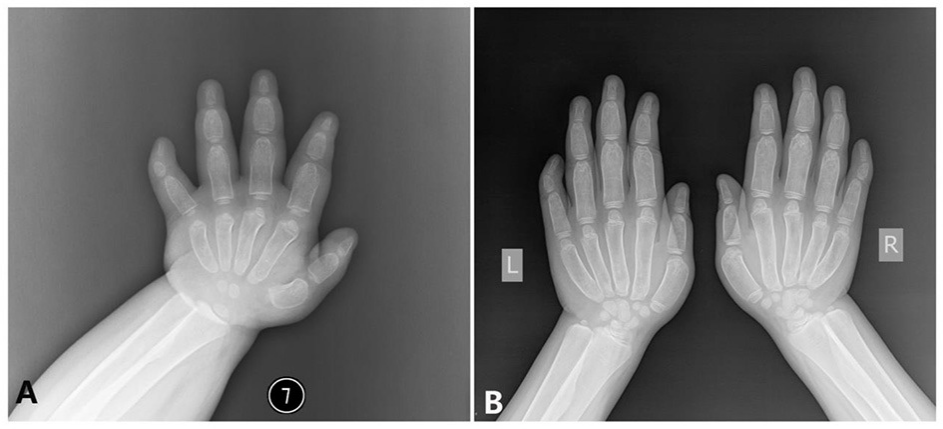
The x-ray radiography of the hands [shows short and wide phalanges and deformable bones; (A) age 1 year and 5 months, (B) age 6 years and 8 months].
